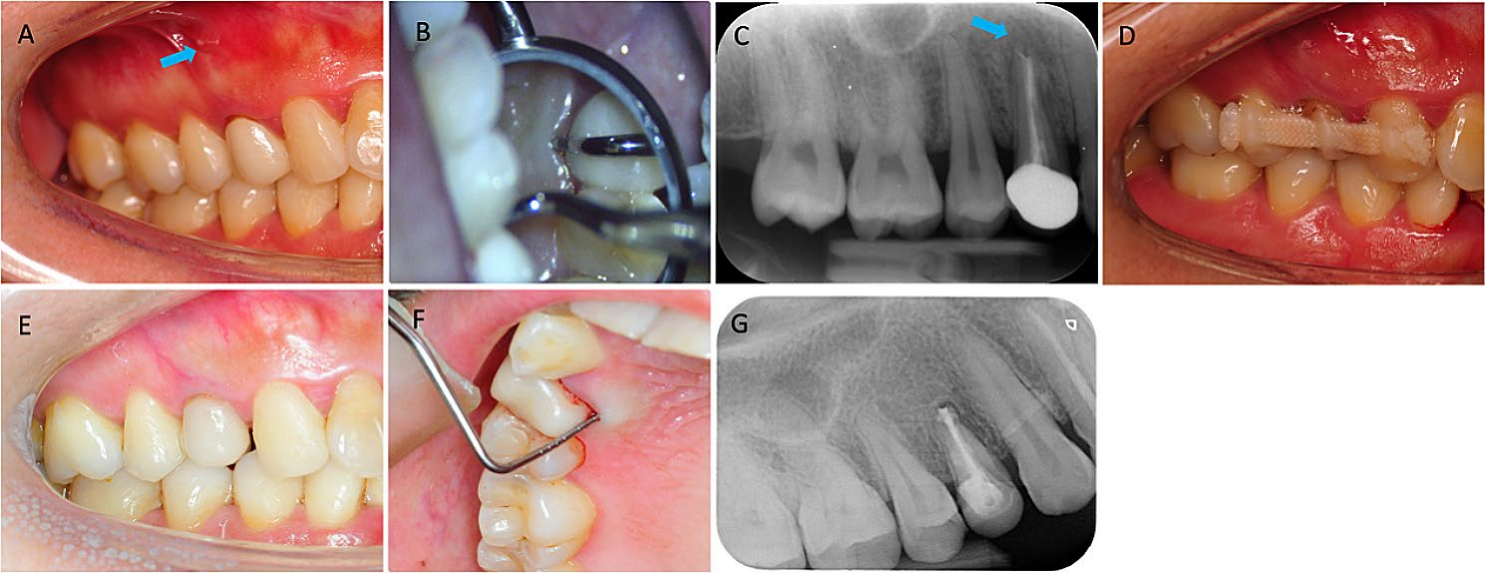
Intra-oral pictures and X-ray images before, after and at 24-month review of surgery. (A) Intra-oral picture before surgery showing a sinus track near the apical area of tooth #14(arrow); (B) Deep narrow isolated pocket on the palatal side of tooth #14;(C) X-ray image before surgery showing a radiolucent area around the apex of tooth #14(arrow); (D) Fixation of tooth #14 after the surgery; (E) Intra-oral picture at 24-month review showing normal gingiva mucosa;(F) Periodontal probing at 24-month review showing about 3 mm probing depth; (G) X-ray image at 24-month review showing the healing of periapical defect
